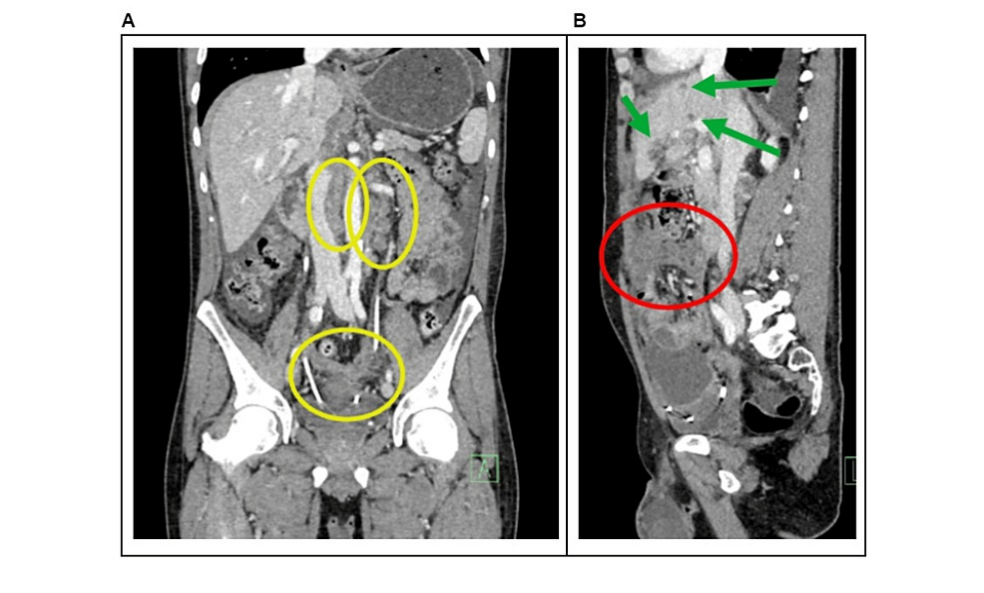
Restaging CT examination at 18 months (venous phase) (A) Anteroposterior reconstruction with the progression of paraaortal malignant lymph nodes (yellow circles). (B) Lateral reconstruction of the abdomen with liver metastases (green arrows) and tumorous mass in the area of the ascending colon (red circle).
